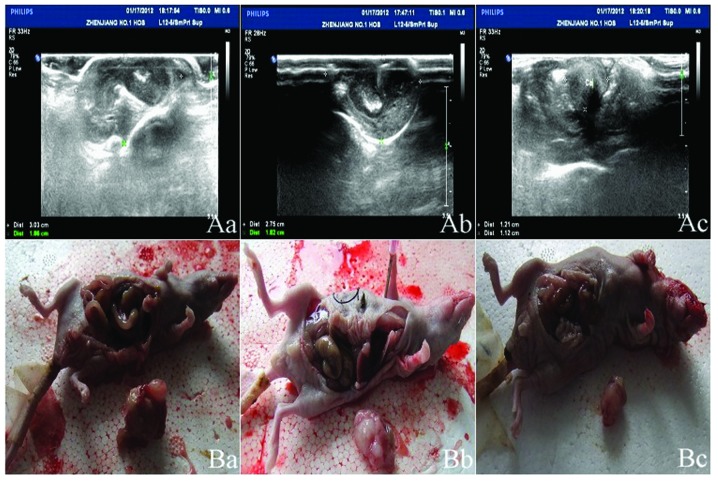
(A) B ultrasound of the abdomen prior to sacrifice and (B) status of the tumor growth of the three groups. (Aa and Ba) Blank, (Ab and Bb) Ad-Lac Z and (Ac and Bc) r-Ad-hIFN-λ1 groups. Following a month of intervention the tumor volume of the r-Ad-hIFN-λ1 group was markedly lower than that of the blank and Ad-Lac Z groups. Ad-Lac Z, adenovirus encoding bacterial β-galactosidase; r-Ad-hIFN-λ1, human interferon-λ1 recombinant adenovirus.
